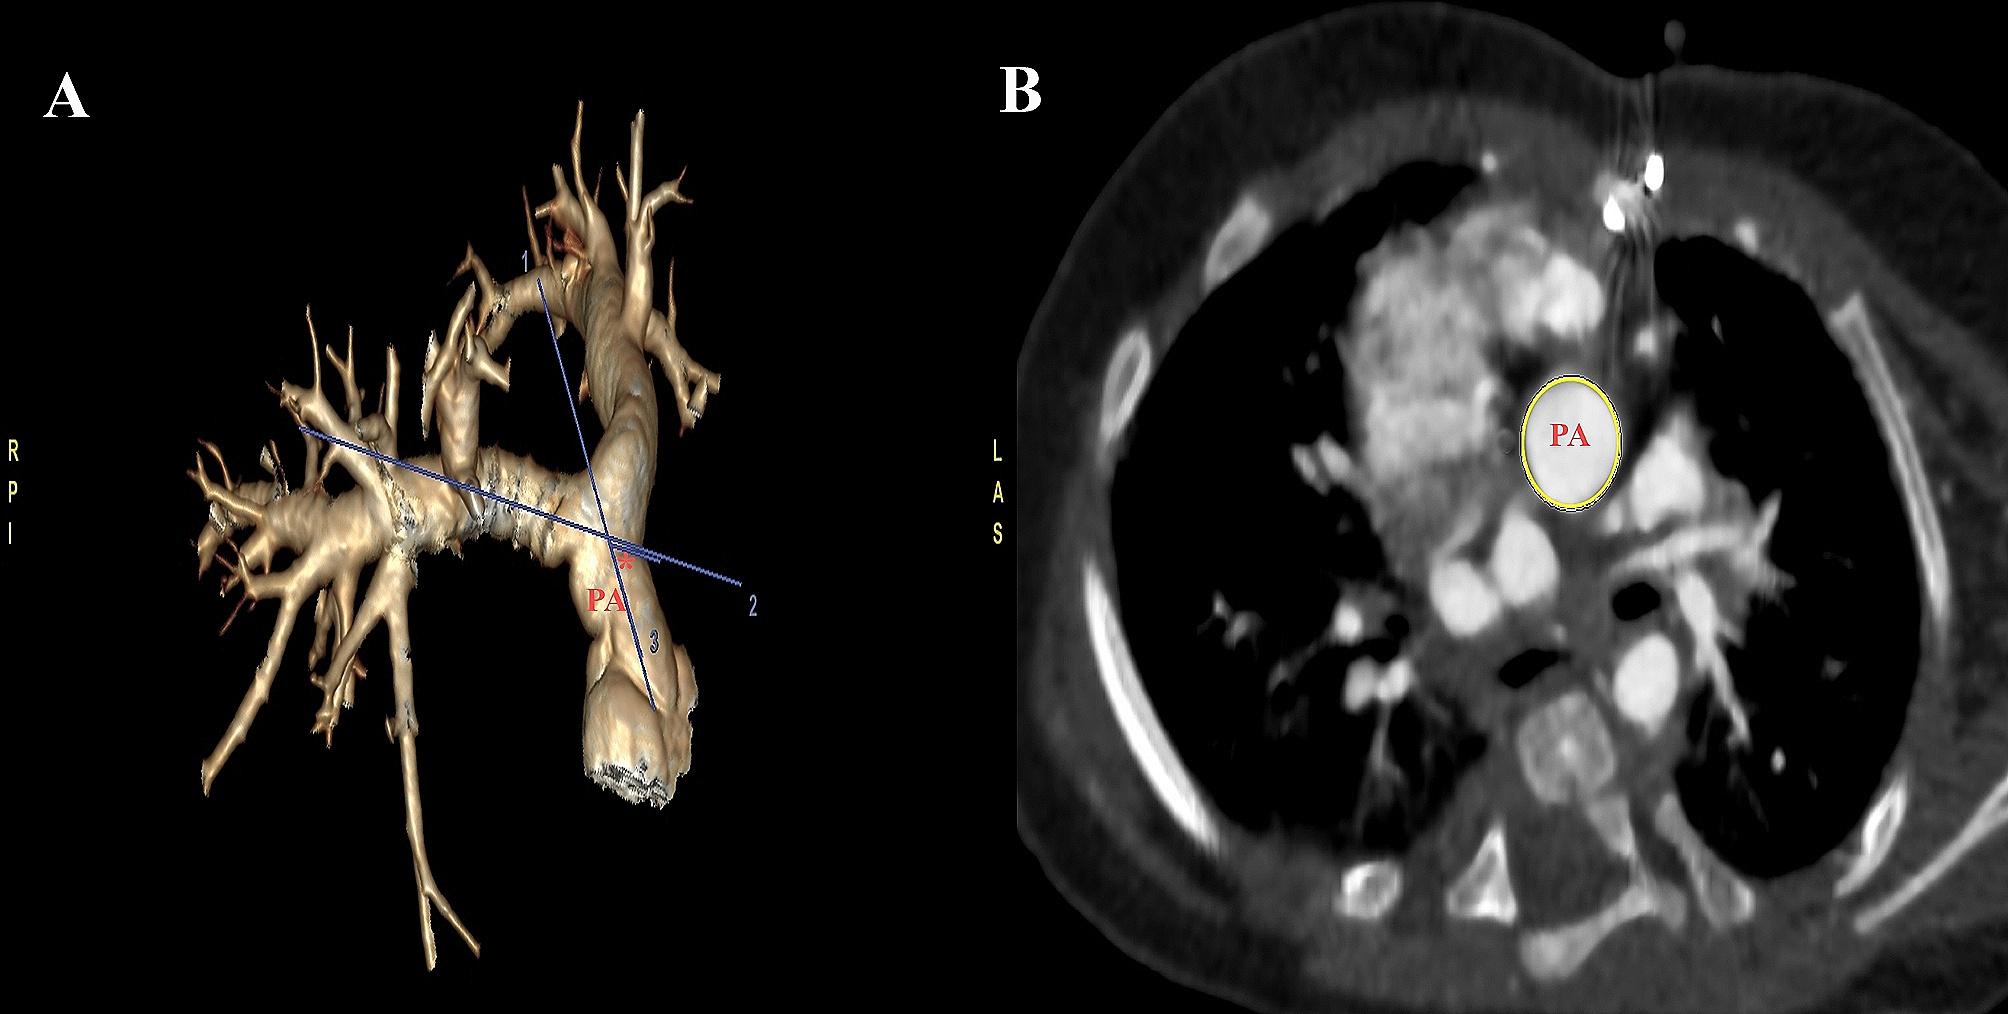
(A) Volume-rendered CT images of the pulmonary arteries showing the pulmonary artery bending angle (red asterisk) as the outer angle between main pulmonary artery axis (axis 1) and branch pulmonary artery axis (axis 2), (B) Axial oblique CT image showing the cross-sectional area of the pulmonary artery (yellow circle). PA: main pulmonary artery
